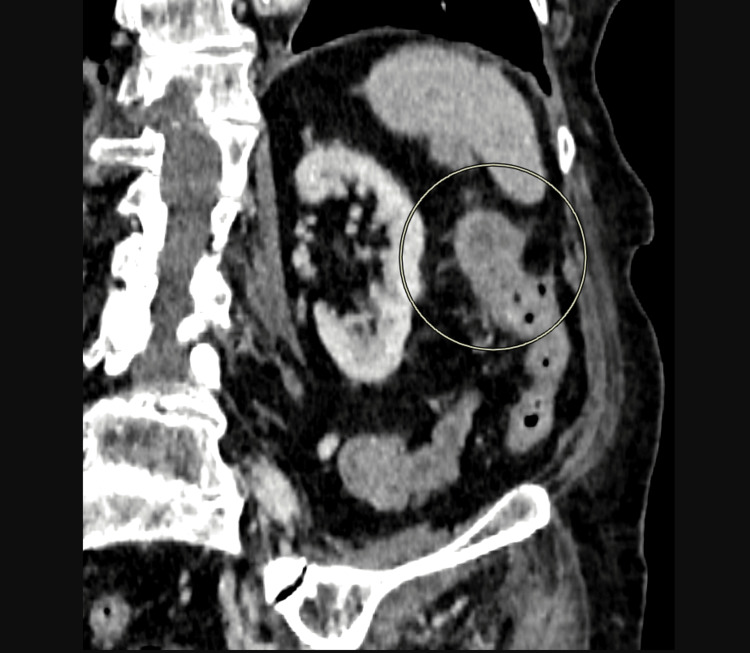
CT scan finding of thickening of sigmoid colon as marked by circle
